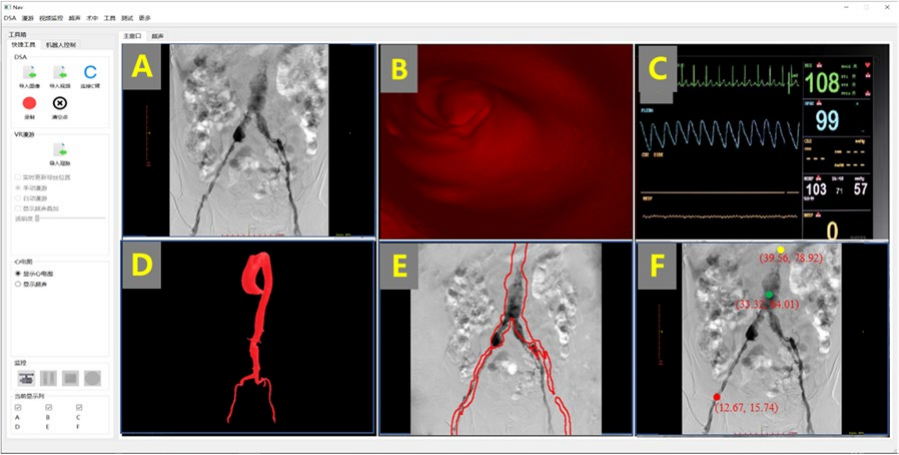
Navigation system for robot assisted MIVI. A was the intraoperative DSA, B demonstrated the internal vascular view, C was intraoperative physiological signal monitoring, D was reconstructed 3D vascular model from CTA, E represented the 2D-3D registration result, F demonstrated positioning of surgical instruments
